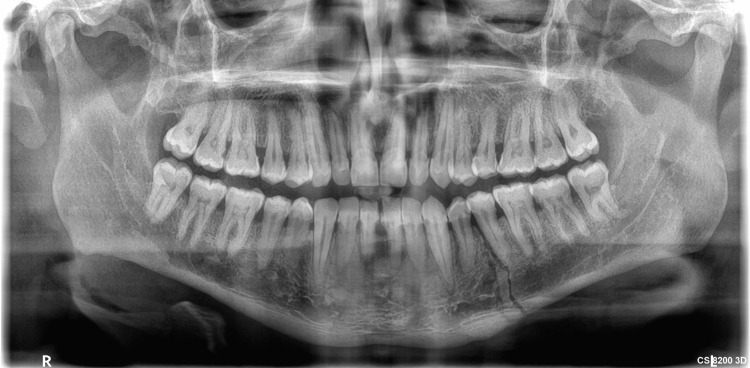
A preoperative orthopantomogram showing an oblique fracture line between teeth 34 and 35, confirming left mandibular body fracture.
